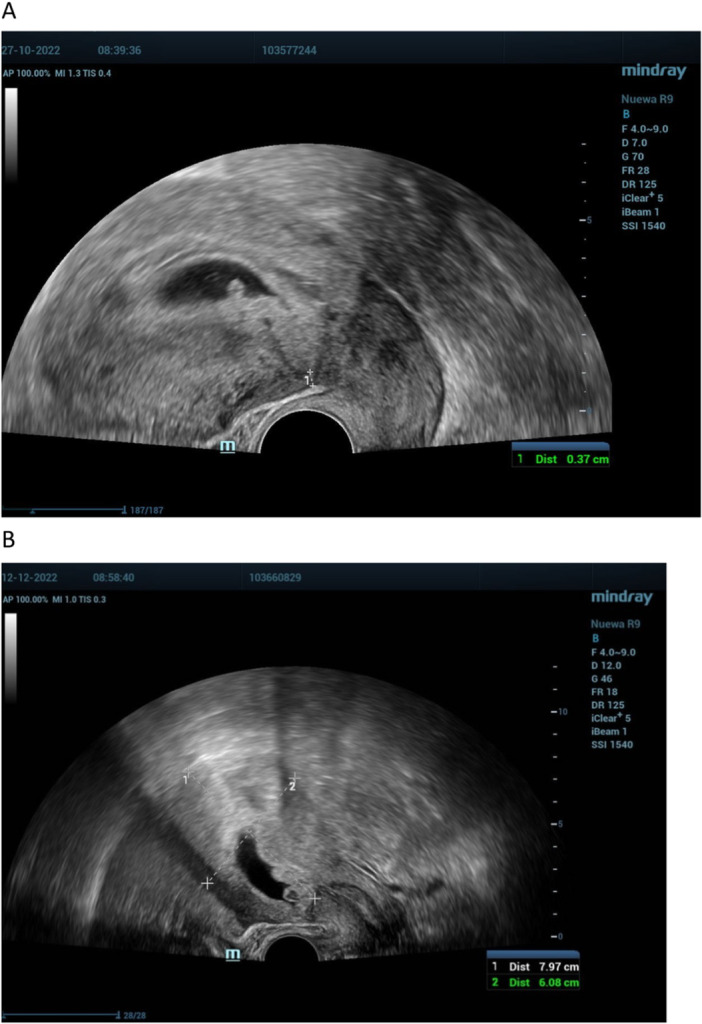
Ultrasound images showing type 1 (A) and type 2 CSP (B).
